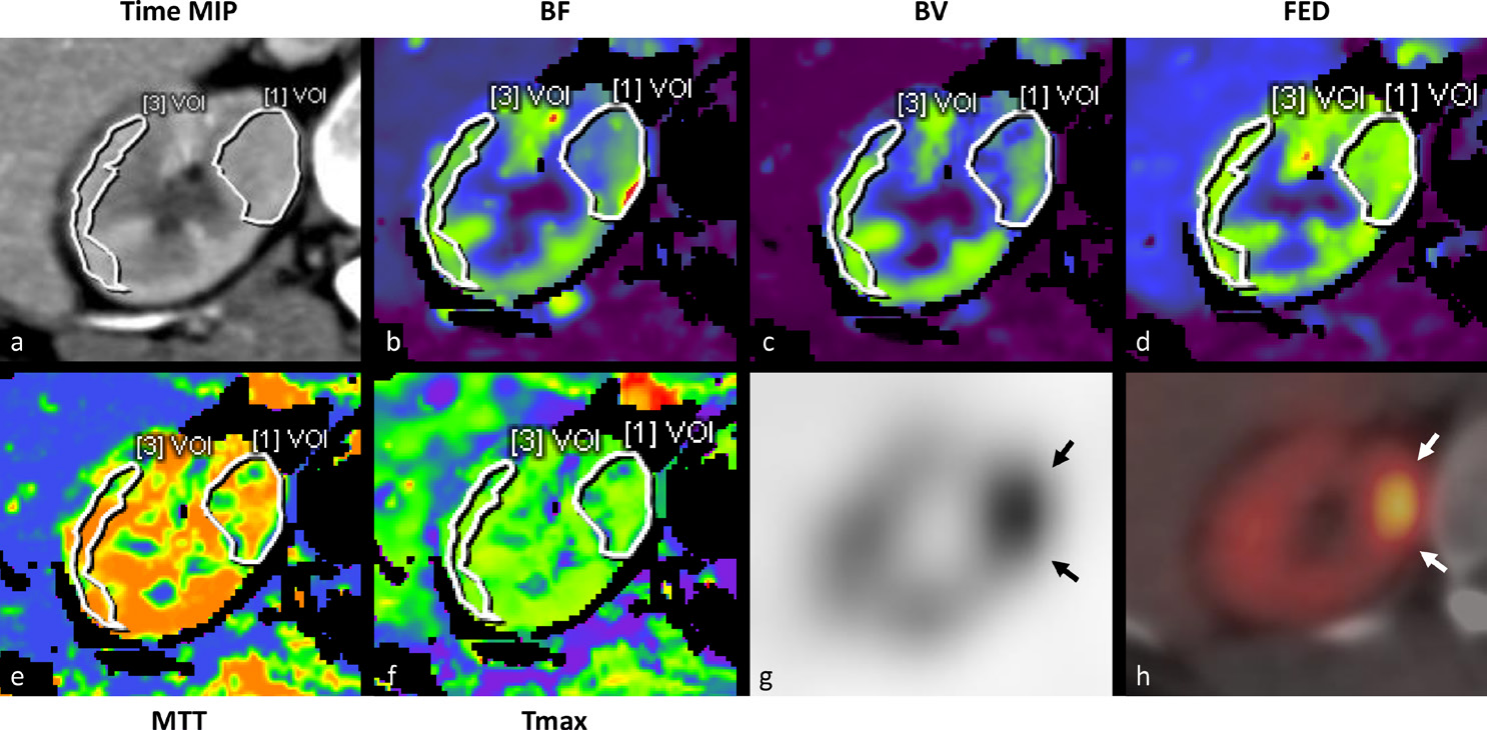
(a–f) Time MIP and parametric maps of BF, BV, FED, MTT, and Tmax of the right kidney. A VOI including the suspect lesion (1) and part of the renal cortex (3) was manually defined in order to calculate the tissue attenuation curve and mean perfusion values. The lesion exhibited lower BF and BV than the renal cortex. On ^99m^Tc-Sestamibi SPECT/CT (g–h), the lesion demonstrated clear focal uptake relative to surrounding renal parenchyma indicating its possible benign character. BF, blood flow; BV, blood volume; FEP, flow extraction product; MIP,maximum intensity projection; MTT, meant transit time; SPECT, single-photon emission computed tomography; Tmax, time to maximum;TTP, time to peak; VOI, volume of interest.
